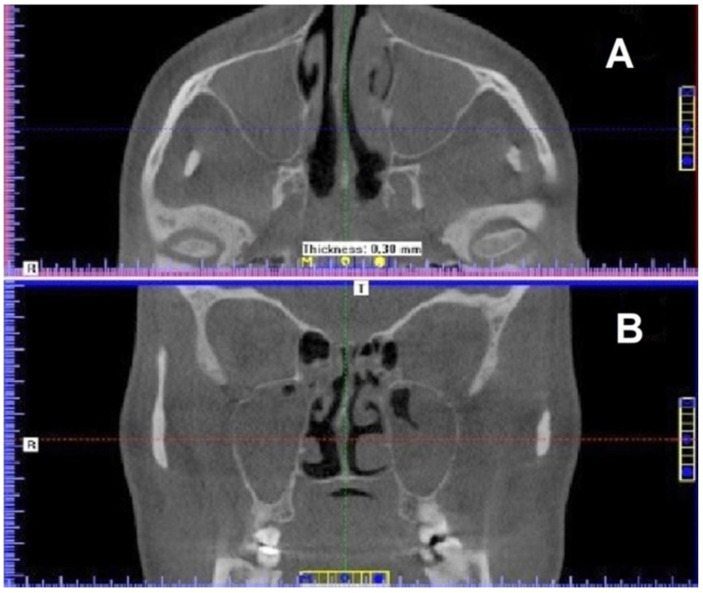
Maxillary sinuses visualized as dark (low-density) areas. (A) axial view; (B) coronal view (CBCT scans).
